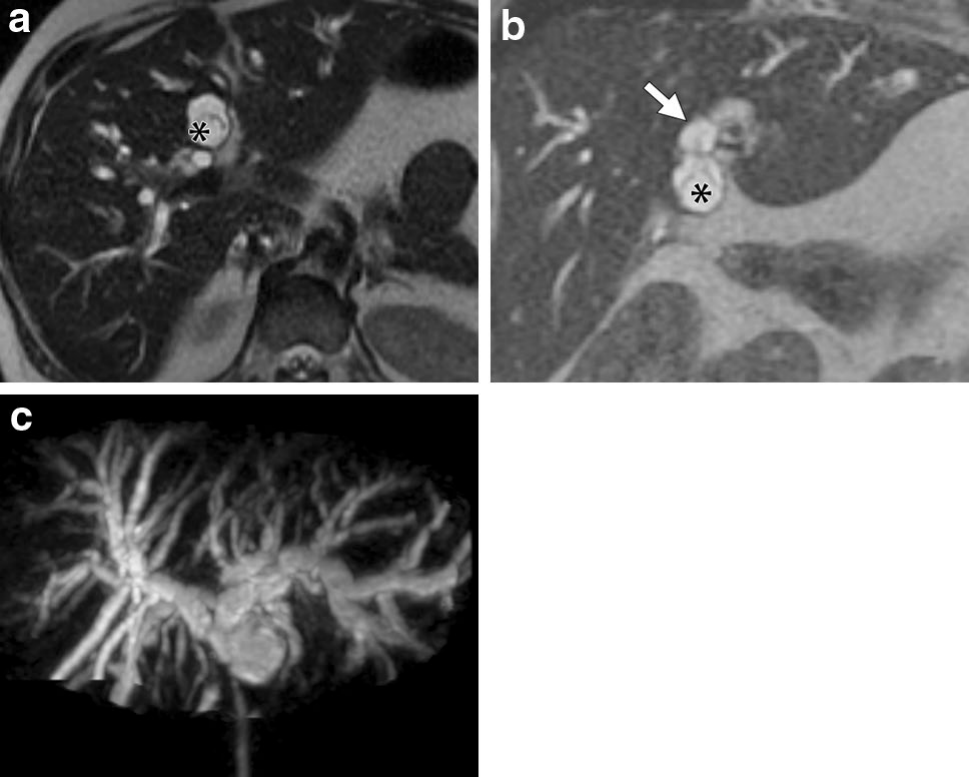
A 56-year-old woman presented with jaundice. She had a multilocular cystic mass 22 mm in diameter in the segment IV of the left lobe of the liver on MRI (a. single shot fast spin echo axial image, asterisk; b. single shot fast spin echo coronal image, asterisks; c. MRCP). Only MRI was available to review. Both readers could tell the biliary prolapse (b, arrow)
